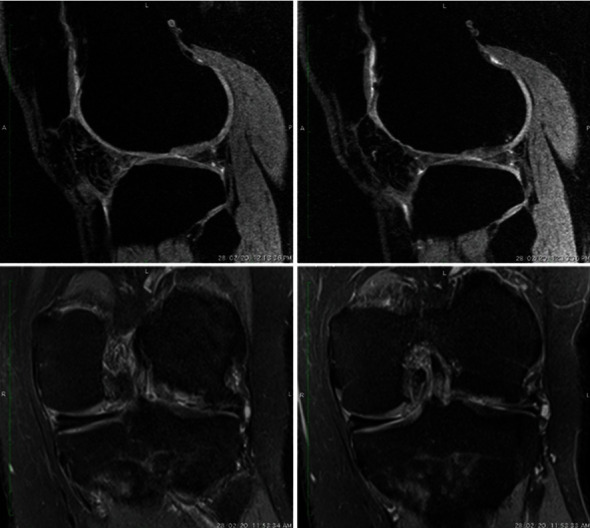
The MRI evaluation posttransplantation showed improvement in cartilage defect.
